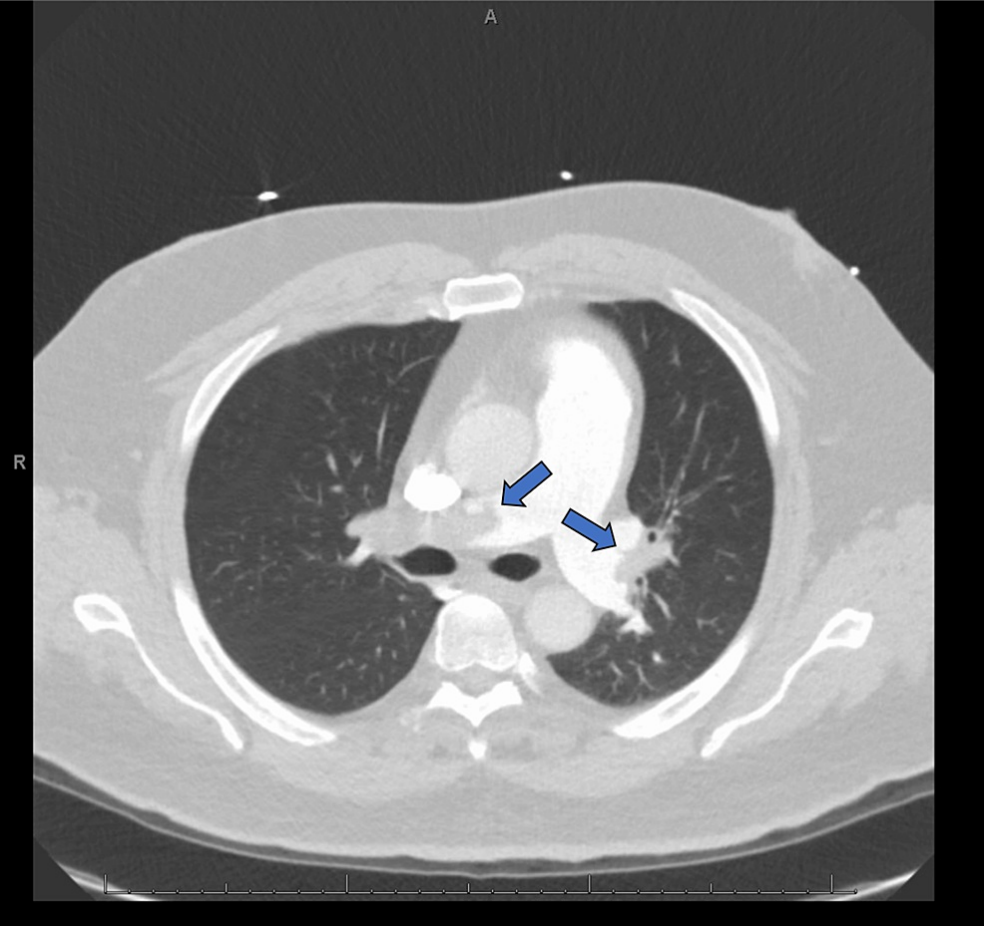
Bilateral central pulmonary emboli on the computed tomography angiography chest
